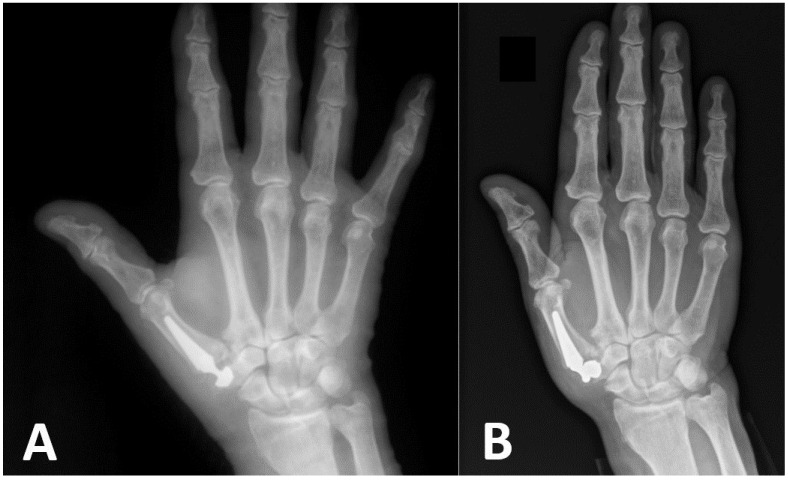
Immediate (A) and two-weeks’ (B) post-operative X-rays showing early cup loosening and dislocation of the prosthesis.
